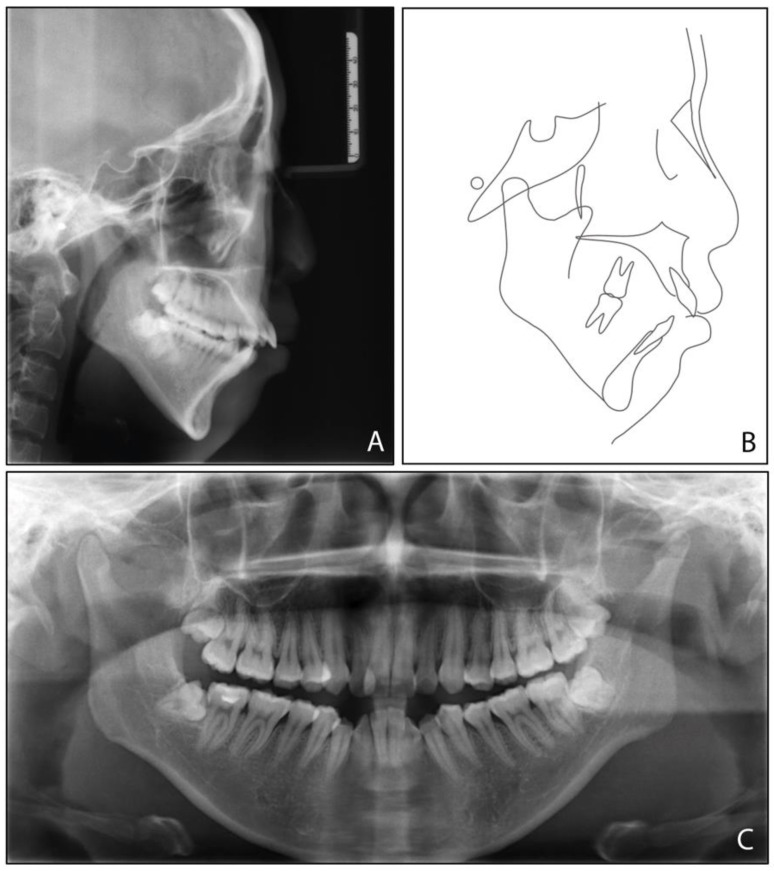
Pretreatment radiographs and tracing: (A) cephalometric radiograph; (B) cephalometric tracing; (C) panoramic radiograph.
